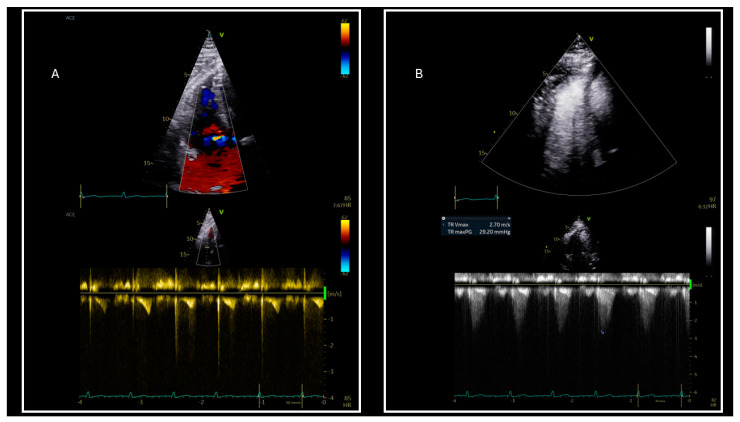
Improvement in accuracy of tricuspid regurgitation (TR) maximum velocity (Vmax) assessment with the use of intravenous contrast enhancing agent. The continuous wave Doppler evaluation of TR Vmax (A) without contrast is suboptimal. (B) TR Vmax with contrast is clearly detected.
